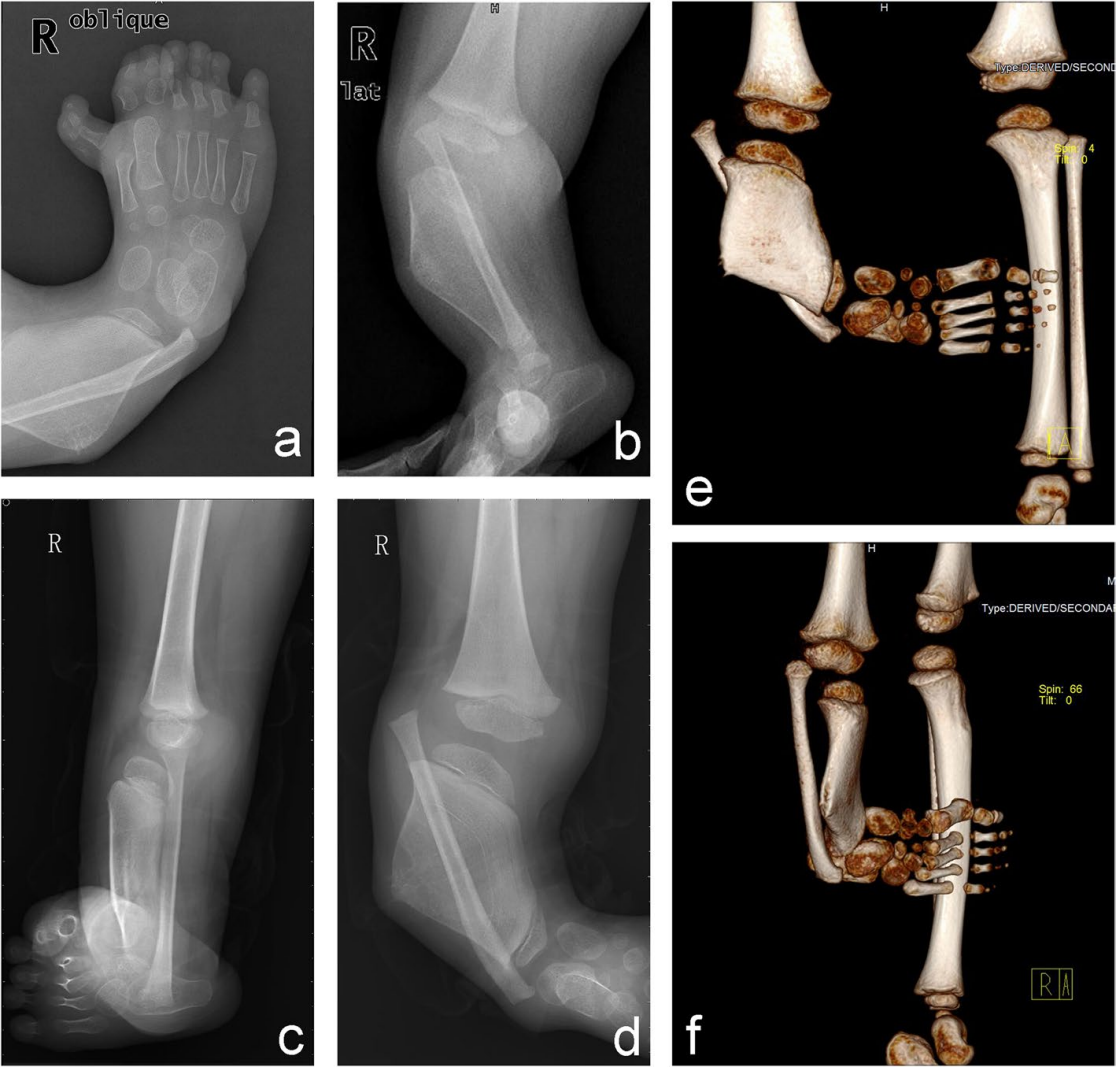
Radiological features of the proband. a X-ray showed polydactyly of the right toes, and a redundant metatarsal forming an articulation with the superfluous toes. b-f X-ray and computed tomography showed shortening, widening and thinning of the tibial stem, slender fibula, as well as dislocation of the and ankle
